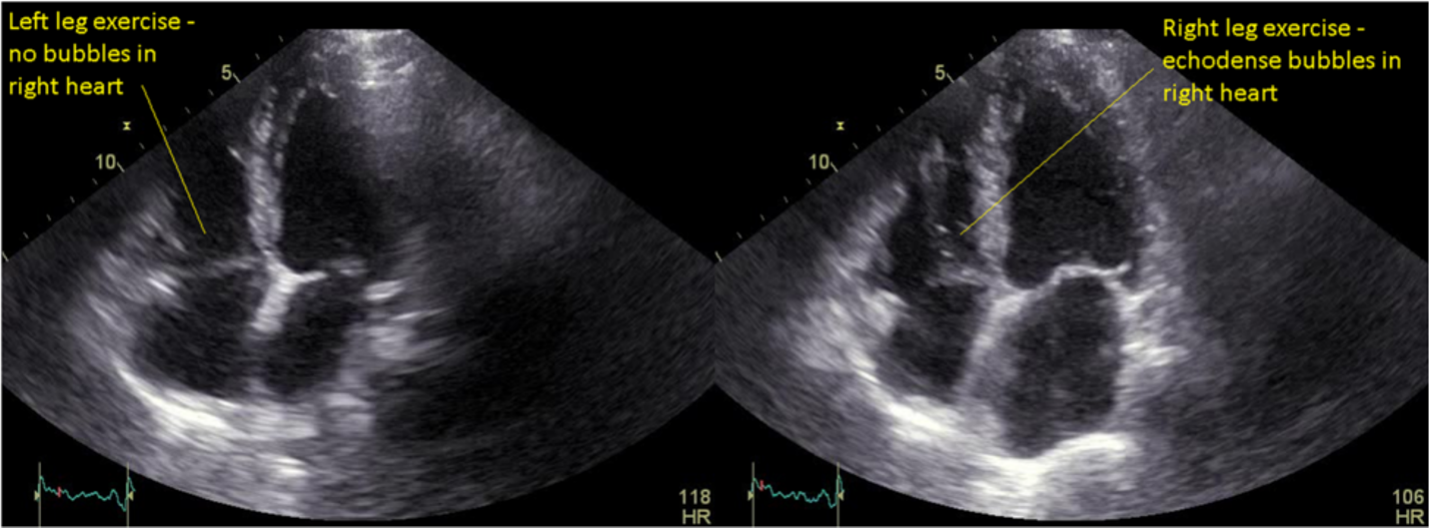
Exertional fat embolism on bicycle stress echocardiography with isolated exercise of the right leg. Apical four-chamber views directly following the previous study (Figure 1), showing no bubbles in the right heart on isolated left leg exercise but a small number of echodense bubbles on isolated right leg exercise. Exercise was performed with a supine bicycle and sequential use of the left and right legs. HR, heart rate.
